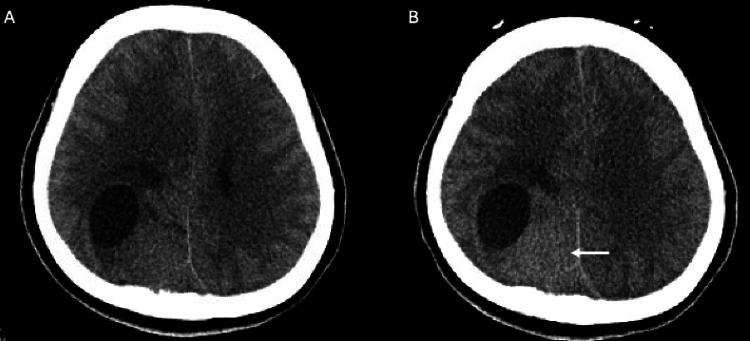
CT findings (A) Non-contrast CT image obtained prior to embolization, demonstrating a tumor with cystic components. (B) CT image obtained four hours after embolization. Although four hours had elapsed, the contrast agent entrapped within the tumor remained stagnant without washout (white arrow).
